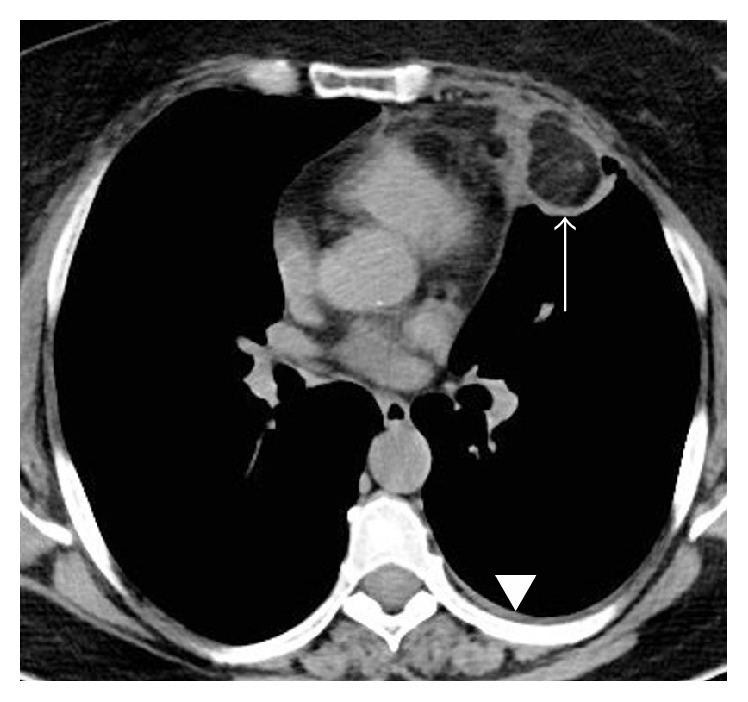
CT scan without contrast enhancement showing a left anterior low-attenuation (−80 HU) pericardial mass with high attenuation strands surrounded by an irregular capsule (arrow) and a small pleural effusion (arrowhead).
